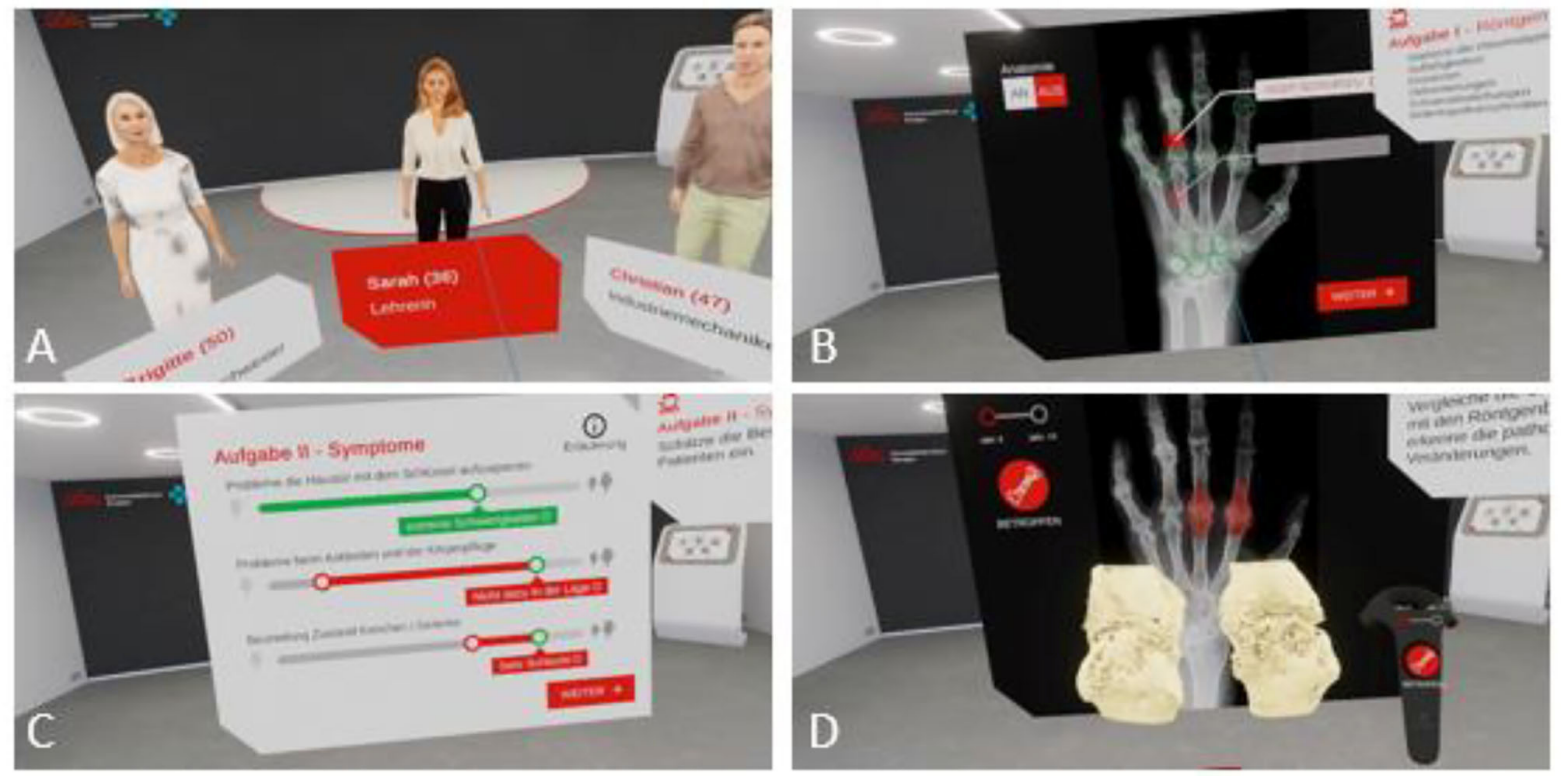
Rheumality-Virtual reality in medical education. Depicted is the welcome scene with the selection of three virtual patients with rheumatoid arthritis and psoriasis arthritis (A). Users can evaluate the X-ray images of these patients for pathological arthritic changes (B) and assess the disease burden of the patients (C). The immersion capability of the 3D visualization of arthritis joints allows in-depth inspection of specific disease-related changes (D).
